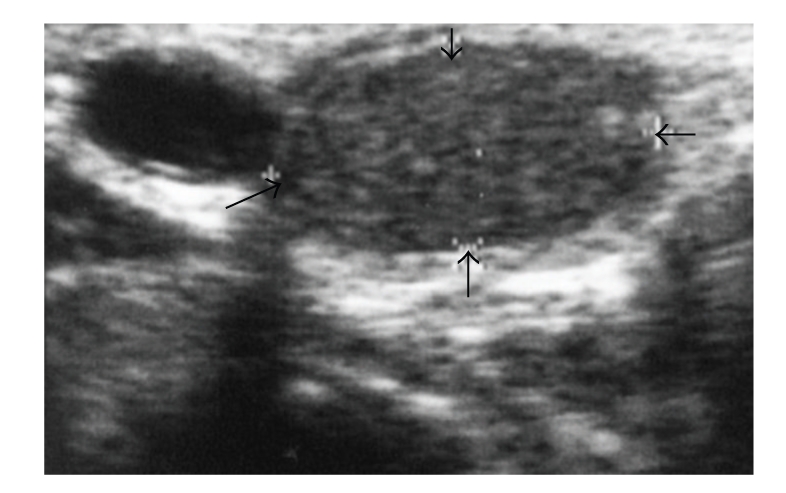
Ultrasound images in the right half of the thyroid gland of an 81-year-old man with squamous cell carcinoma on the right side of the tongue and metastasis in one of the superior internal jugular lymph nodes. A 2.6-cm echogenic mass (arrows) in the right half of the thyroid gland is shown.
